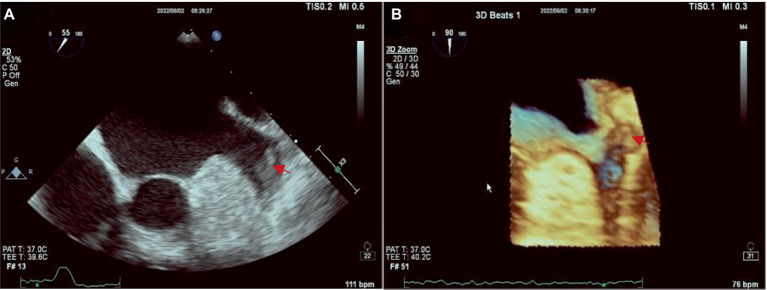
(A) The tip of LAA was a fresh thrombus (red arrow) at TEE. (B) Image of 3D ultrasound.
